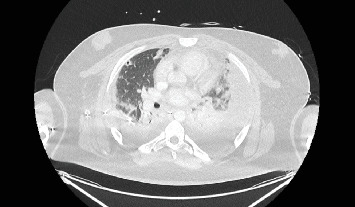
Transverse computed tomography thorax on Day 6 after right intercostal drain insertion on Day 4 demonstrating resolution of pneumothoraces, right intercostal chest drain, bilateral pleural effusions with compressive atelectasis, multifocal consolidation, many demonstrating cavitation.
